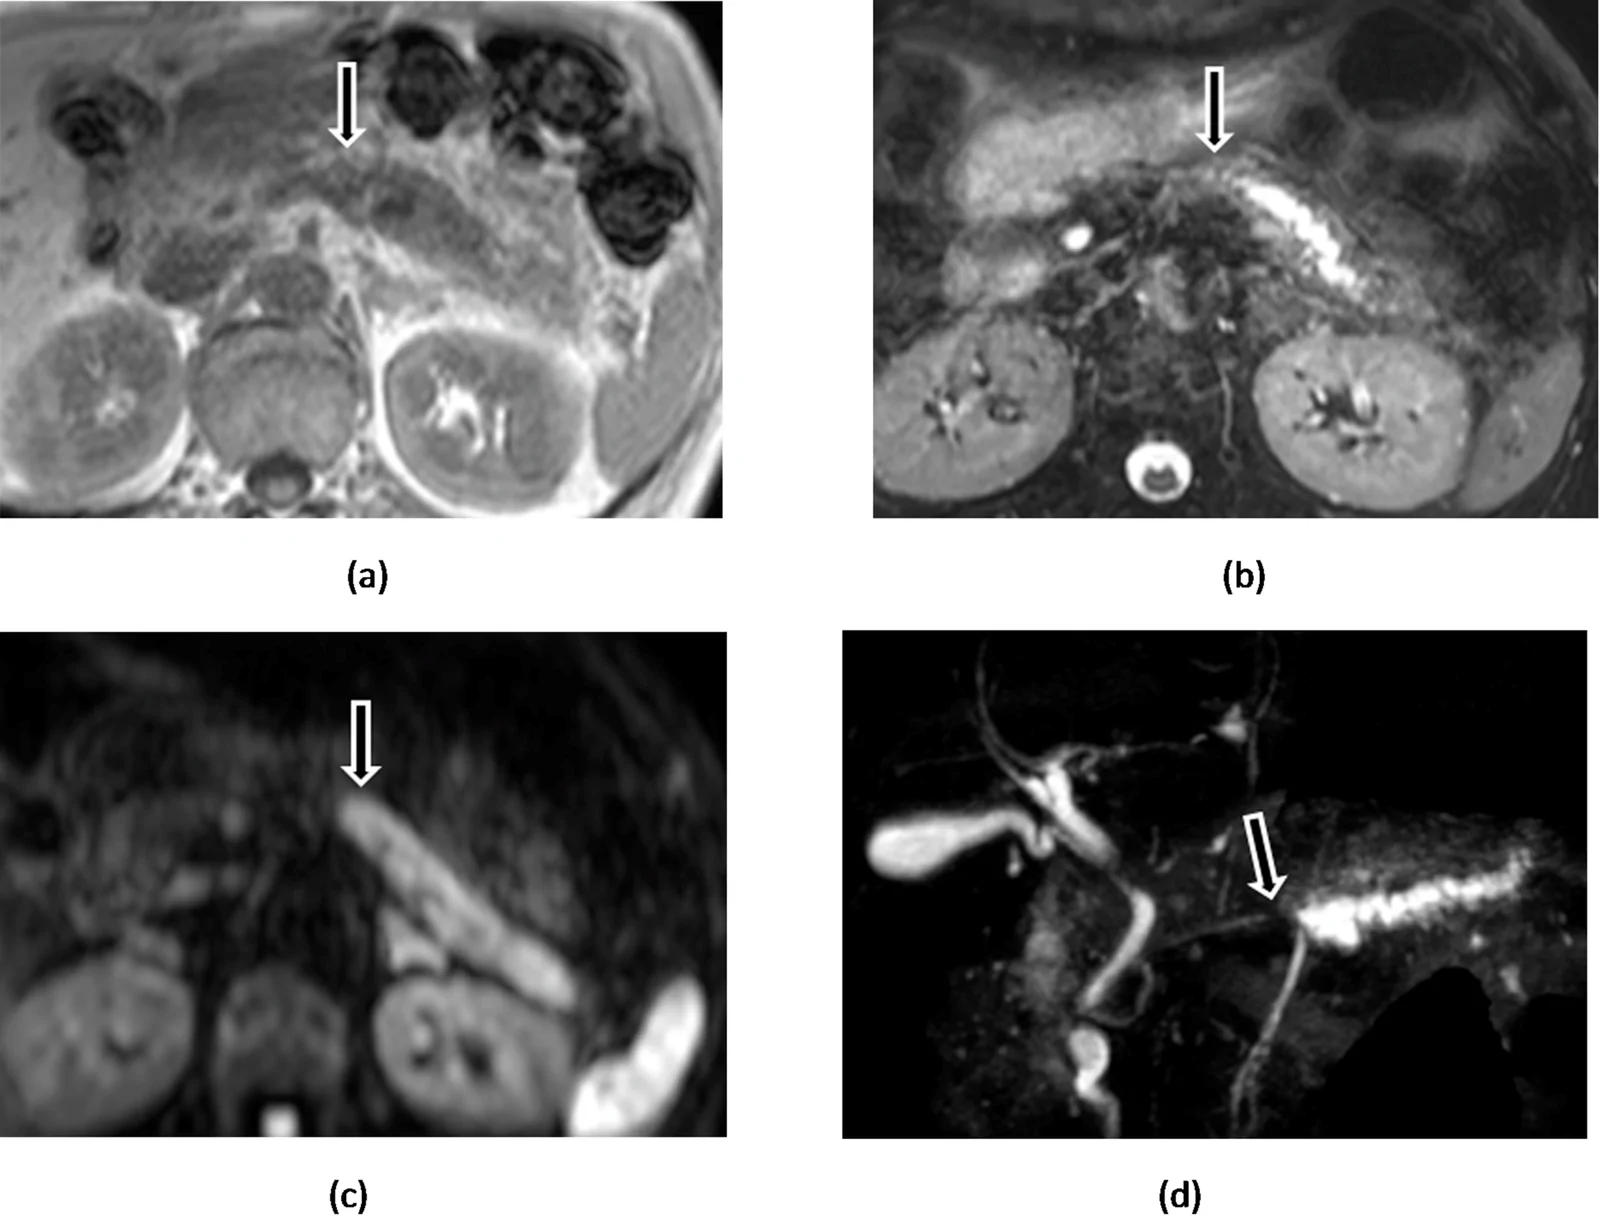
A 73-year-old female patient with pancreatic ductal adenocarcinoma in the pancreatic body measuring 10 mm. The tumor (arrow) is ill-demarcated, as indicated by hyperintense signal changes in the pancreatic tail on T1-weighted (a), fat-saturated T2-weighted (b), and diffusion-weighted (c) images. Stenosis of the main pancreatic duct with upstream ductal dilatation is clearly visible on magnetic resonance cholangiopancreatography (d)
